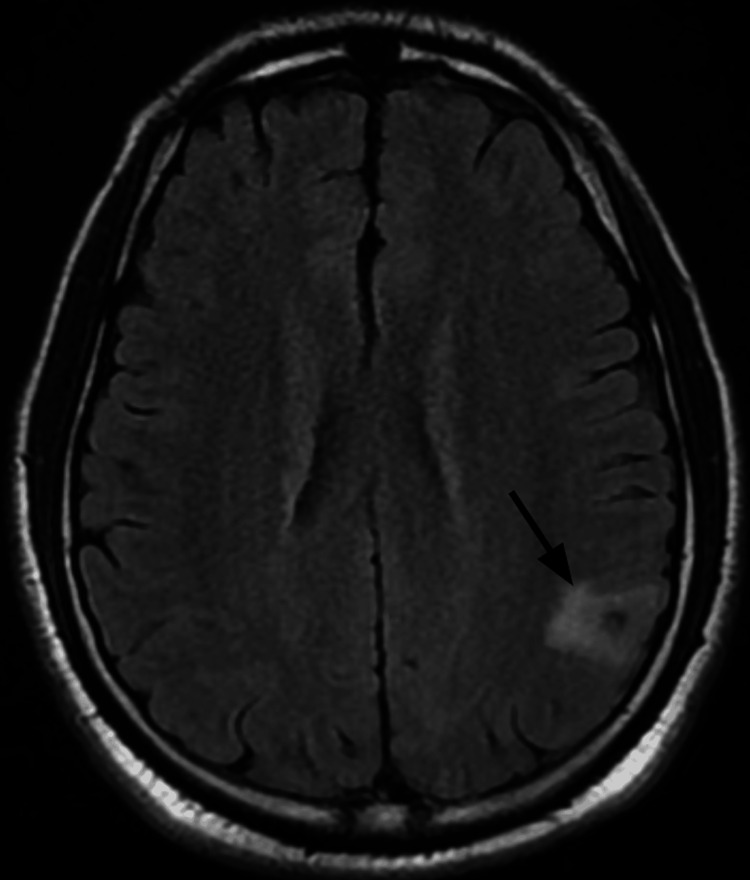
T2 FLAIR MRI of the brain reveals a second lesion in the left parietal lobe surrounded by vasogenic edema FLAIR: Fluid-attenuated inversion recovery
